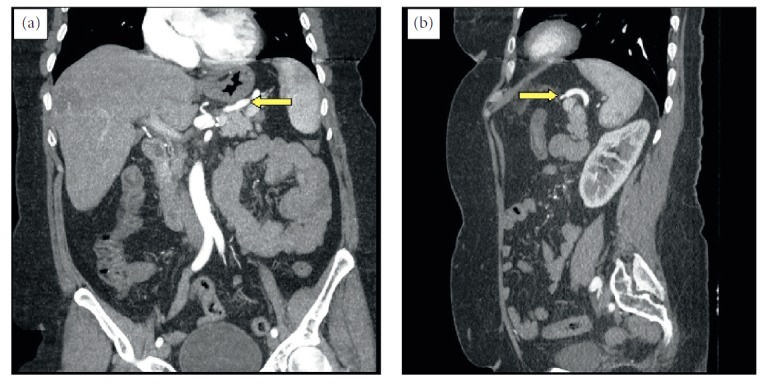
Computed tomographic image taken six months after surgery.
